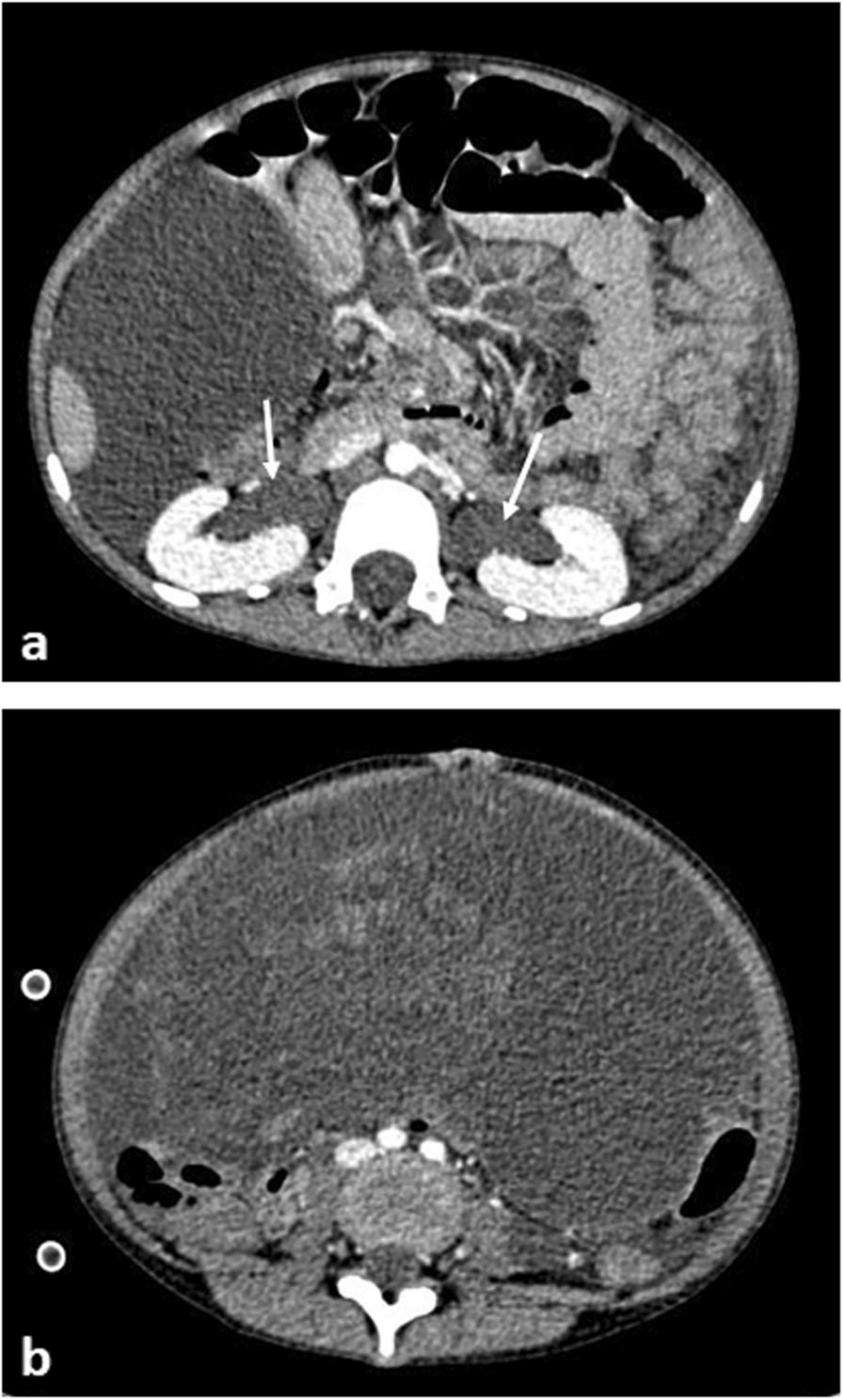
An 8-year-old girl with large Sertoli Leydig cell ovarian tumour arising from the right ovary. a Axial contrast-enhanced CT of the upper abdomen demonstrates bilateral hydronephrosis (white arrows) secondary to (b) the large intra-abdominal mass, which was compressing the distal ureters (not visible in image)
